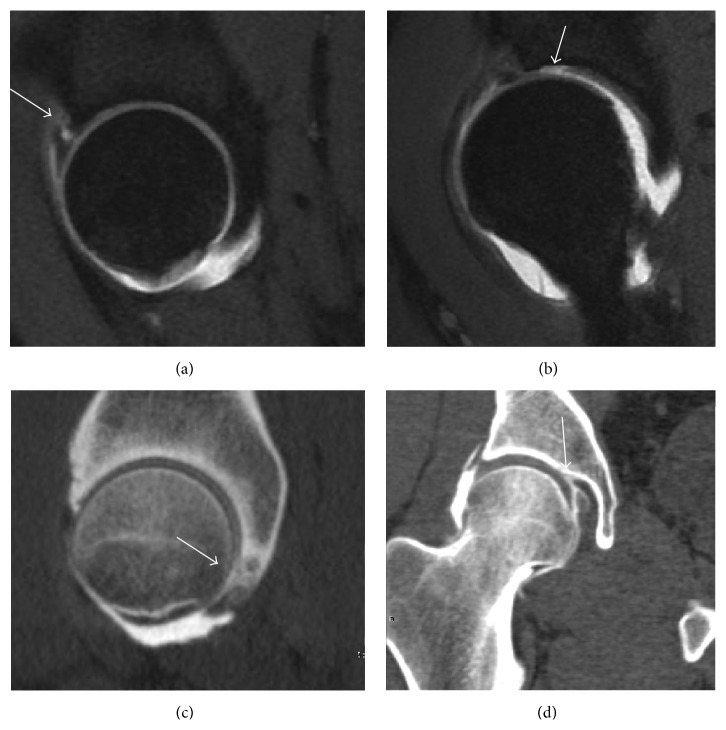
Sagittal T1 weighted images showing anterosuperior labral tear (a) and chondral lesion (b). Sagittal CT-arthrography showing posteroinferior chondral injury (c) and coronal CT-arthrography (d) showing ligamentum teres tear.
